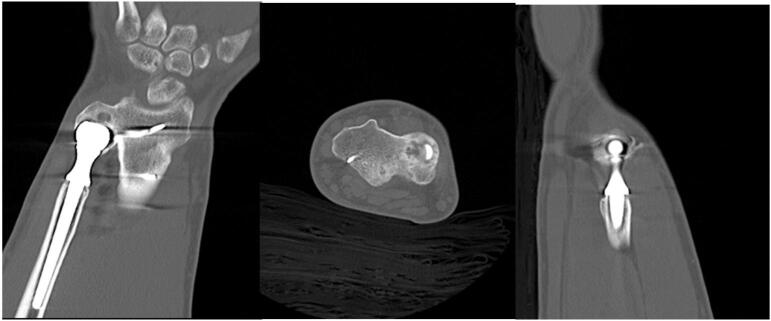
Coronal, axial, and sagittal cuts from the wrist computed tomography (CT) scan showing the pseudo-arthrosis of the distal ulna with the radius and the well fixed ulnar stem.
